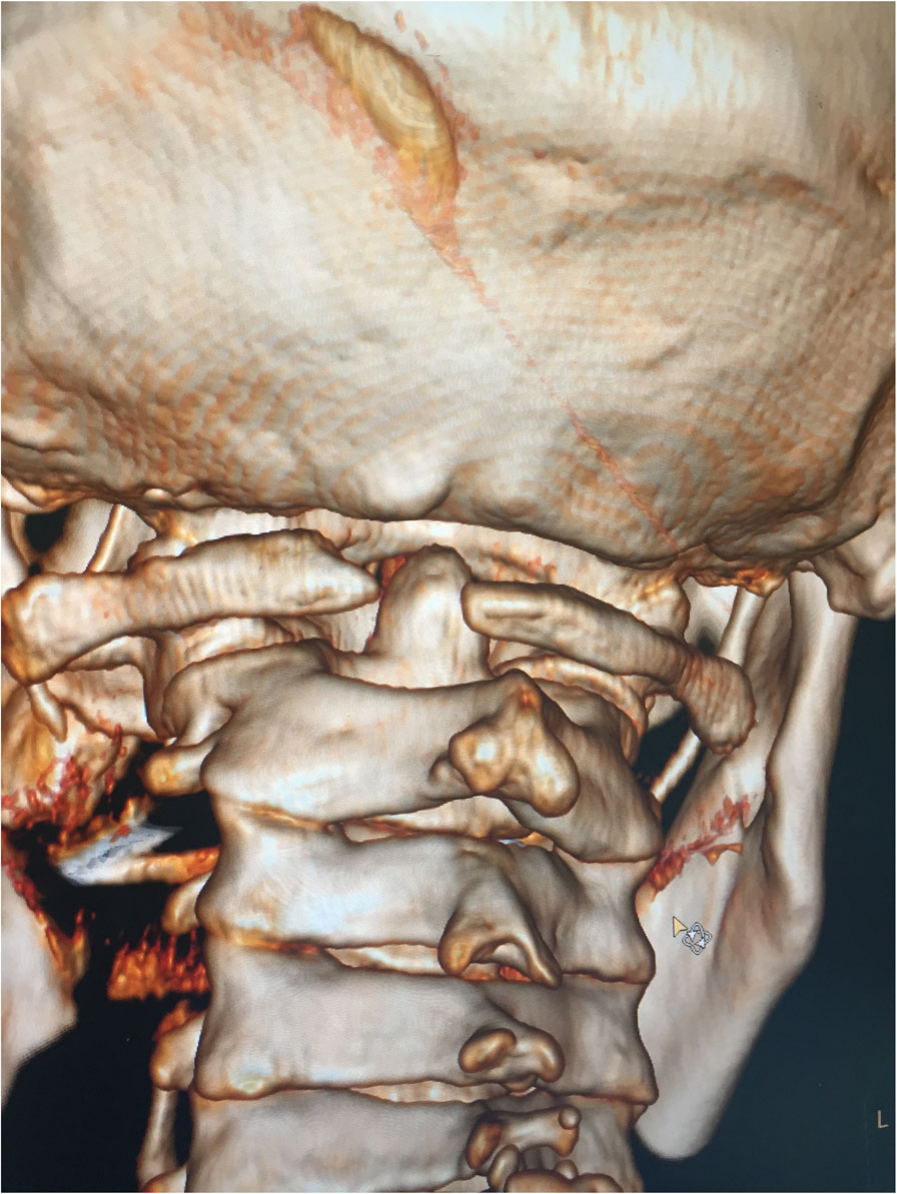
The left side of the posterior arch was revealed as the site of defect on CT 3-dimensional imaging
